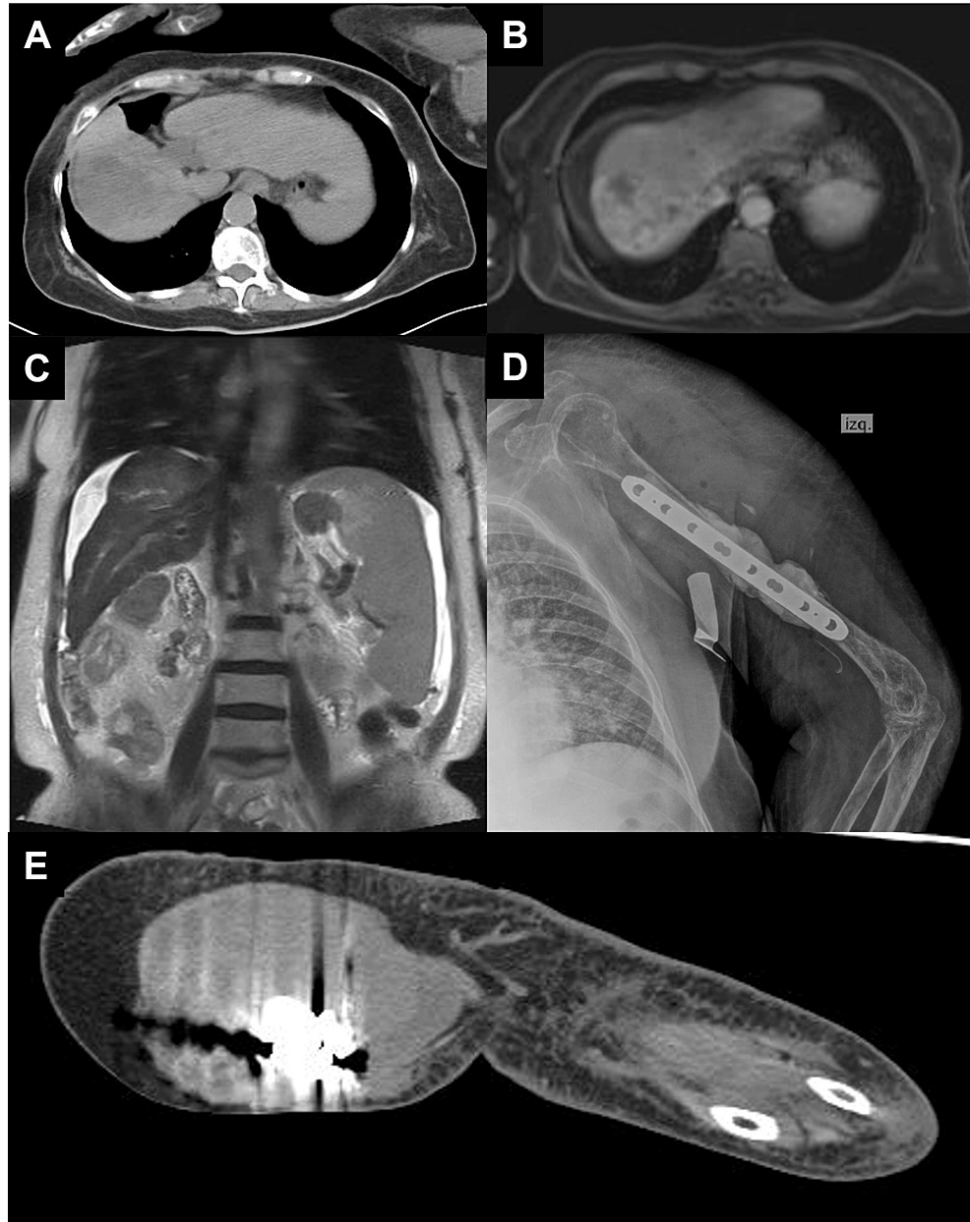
Radiologic findings (A-C) An 85x65 mm heterogeneous, solid mass with a wedge-shaped morphology located in the hepatic segments VII and VIII. It partially surrounds the right suprahepatic vein near the inferior vena cava, without infiltration of its lumen. Splenomegaly is also appreciated. (D) Left upper arm X-ray showing osteosynthesis of humerus shaft with fracture callus. (E) In the axial plane, there is a neoformative process in the distal-middle third of the left arm that measures 10x10 cm. (A, E: Multislice spiral CT without contrast) (B, C: Contrast-enhanced MRI).
